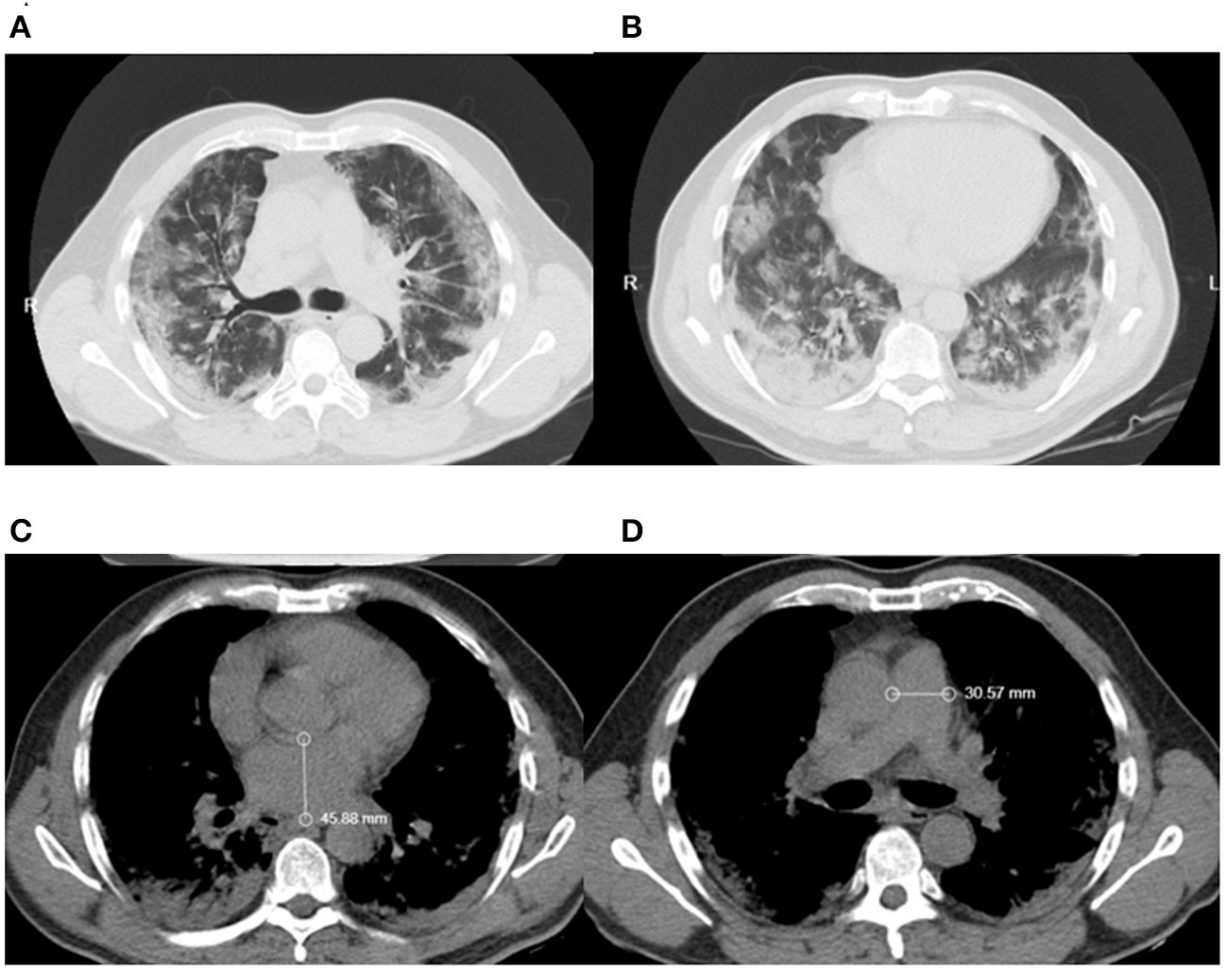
Patients chest CT showing (A) vascular enhancement sign (B) ground-glass opacities (C) left atrium diameter (D) pulmonary artery trunk diameter.
